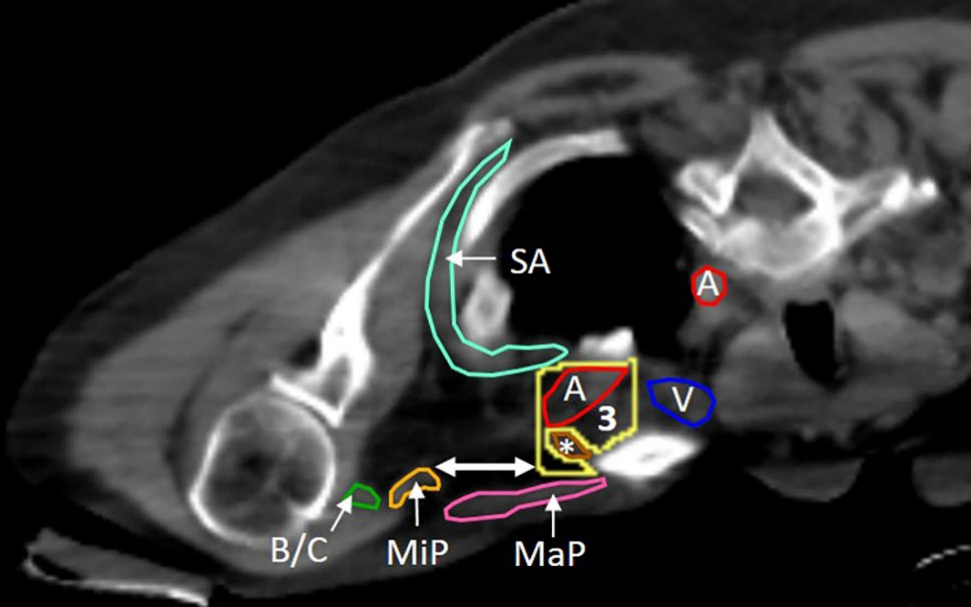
Adaptations to level III borders indicated on patient CT scan taken in PCP. The figure shows the first cranial slice where the axillary/subclavian artery first crosses both the lateral border of the first rib and the clavicle. In the most cranial slices of level III, the minor pectoral muscle can still be located (very laterally) close to its insertion on the coracoid process of the scapula. To spare the (up to 30 mm) margin indicated by the double arrow, the lateral border is taken at the lateral edge of the axillary artery until the artery crosses the medial edge of the minor pectoral muscle. Also note that the CTV excludes the subclavius muscle. Turquoise (SA) = serratus anterior muscle, red (A) = common carotid artery, or axillary/subclavian artery, yellow (3) = level III, blue (V) = subclavian vein, brown (*) = subclavius muscle, orange (MiP) = minor pectoral muscle, dark green (B/C) = biceps/coracobrachial muscle bundle, pink (MaP) = major pectoral muscle.
